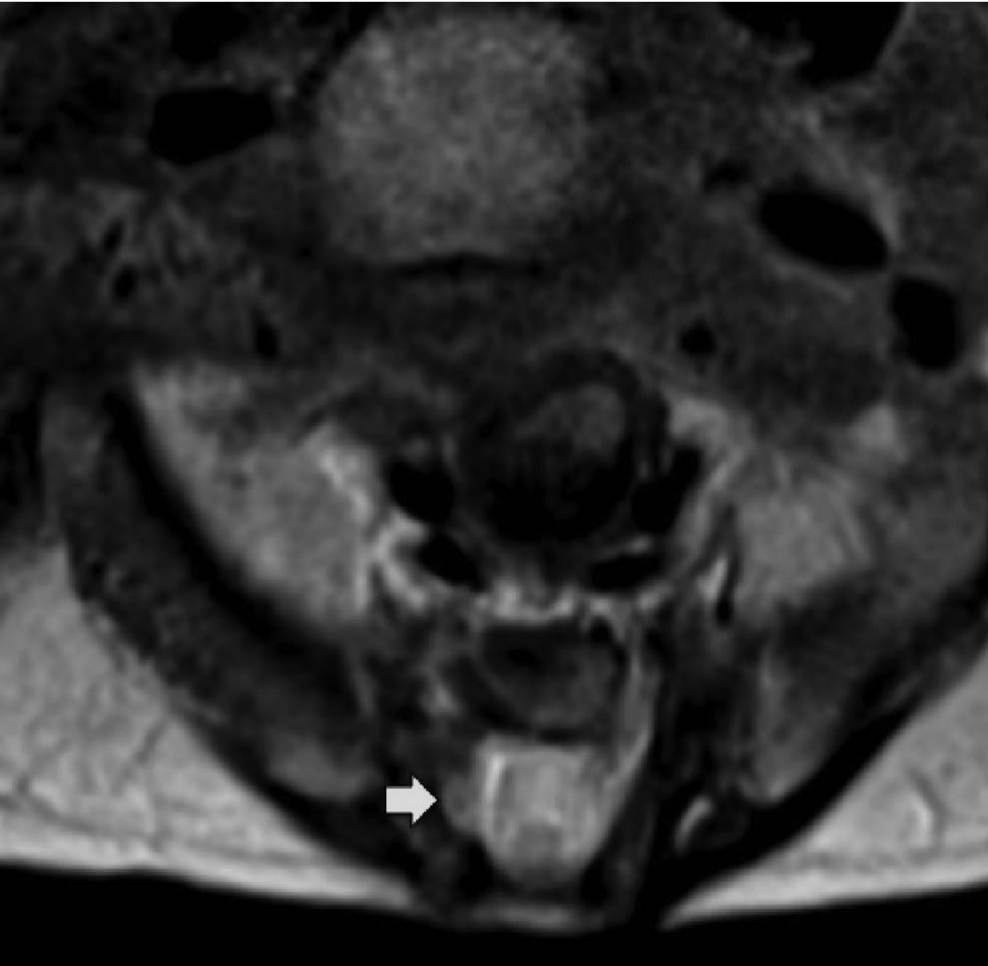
Axial T2-weighted magnetic resonance imaging at the level of the sacrum shows the thecal sac with expansion and posterior cord tethering as a result of posterior spinal dysraphism (arrow).
